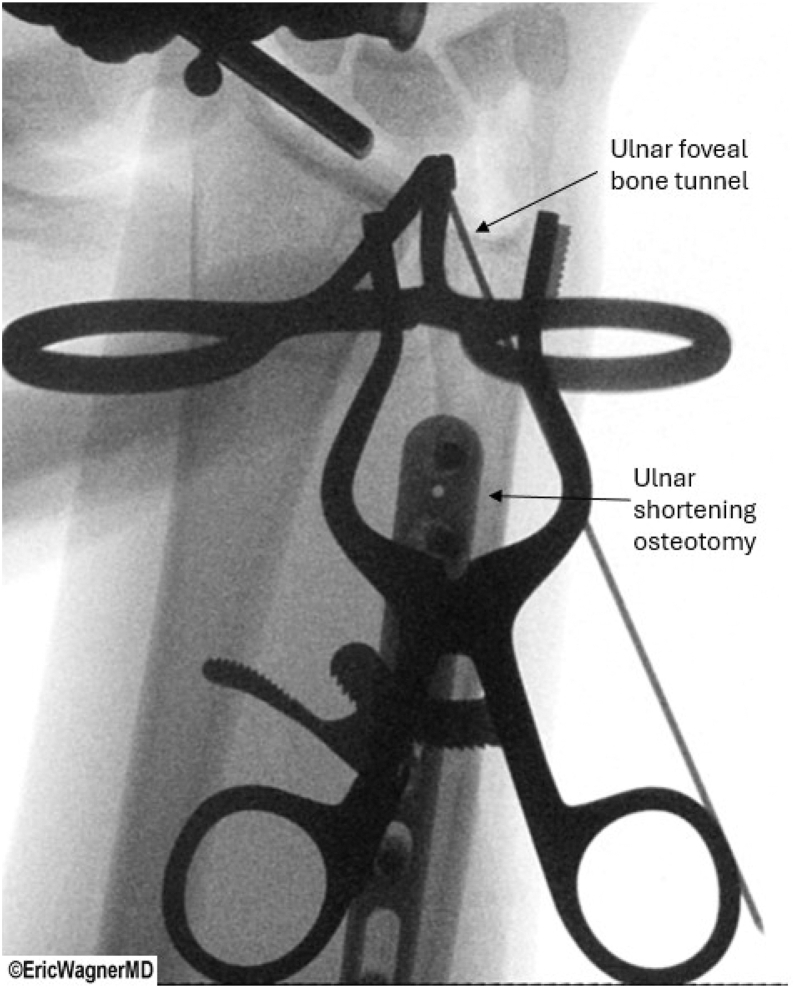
Concomitant ulnar shortening osteotomy. Intraoperative X-ray of a patient who underwent ulnar shortening osteotomy performed with triangular fibrocartilage complex reconstruction in biomechanically disadvantaged distal radioulnar joints (DRUJs) (e.g., positive ulnar variance). The procedure tightens ulnocarpal ligaments and offloads the reconstruction, showing the osteotomy site and fixation to restore DRUJ congruity. The patient is positioned supine in a wrist traction tower, and this is the right wrist.
